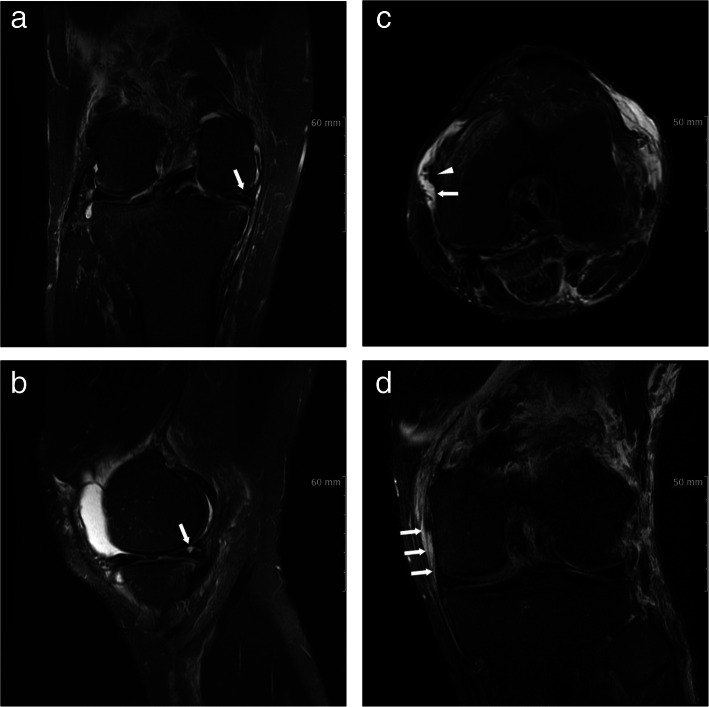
Magnetic resonance image of posteromedial corner injury. a Coronal fat-suppressed image of medial meniscus, showing complex tear of posterior horn (*arrow*). b Sagittal fat-suppressed image of medial meniscus, showing complex tear of posterior horn (*arrow*). c Axial fat-suppressed image of injury to the posterior oblique ligament (POL), showing edema around disrupted POL (*arrow*) and a normal femoral insertion of medial collateral ligament (*arrowhead*). d Coronal fat-suppressed image of injury to POL (*arrows*)
